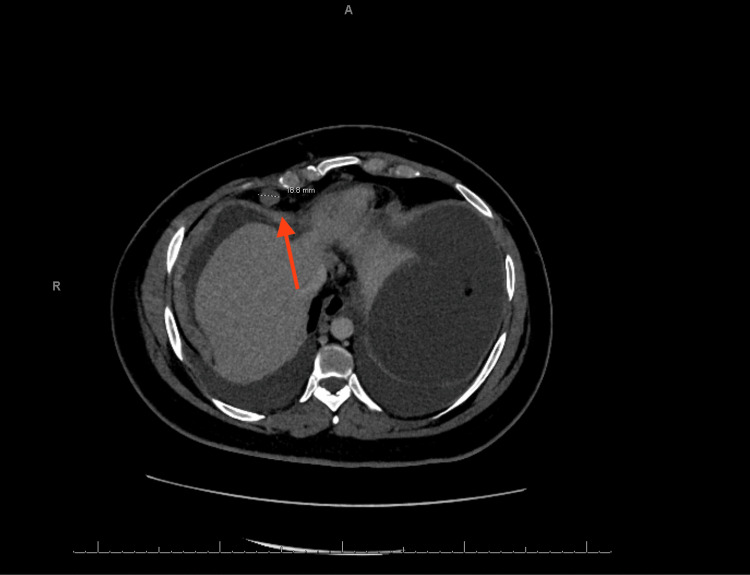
CT scan showing right cardiophrenic lymphadenopathy. The red arrow indicates a 19 mm lymphadenopathy.
